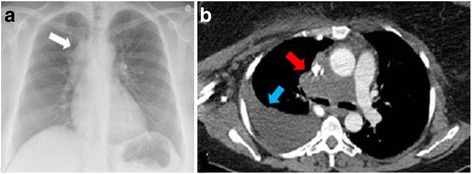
a Chest X-ray showing widening of anterosuperior mediastinum (*white arrow*). b Contrast-enhanced chest computed tomography scan showing a mediastinal mass with right-sided pleural effusion (*blue arrow*). The mass measures 78 by 40 mm and causes compression of the superior vena cava and pulmonary artery trunk (*red arrow*)
